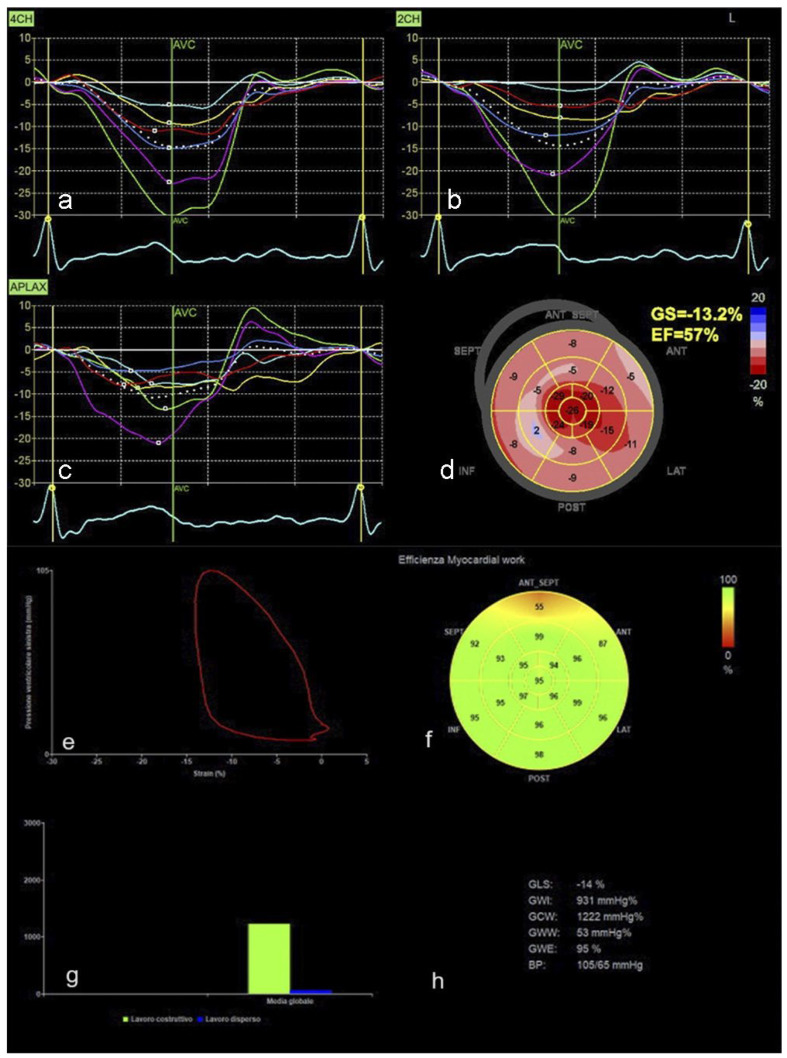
Global longitudinal strain (GLS) and GLS-based myocardial work (MW) software (General Electrics, Boston, MA, USA) by 2D echocardiography in a heart transplanted patient. Results were reported in tables, graphs or plots. GLS is calculated by three apical views, generating 6 plots for each view (a–c); GLS can be also visualised in a bull’s eye plot, displaying 17 segments (d); Left ventricle (LV) myocardial work is based on pressure–strain loop (e); the software can calculate global MW and segmental MW, in the latter case, it can be visualised in a 17 segments bull’s eye (f); segmental analysis allows to study MW efficacy: constructive (green bar) and wasted (blue bar) global work. These data can be displayed in graphs (g); or tables (h).
